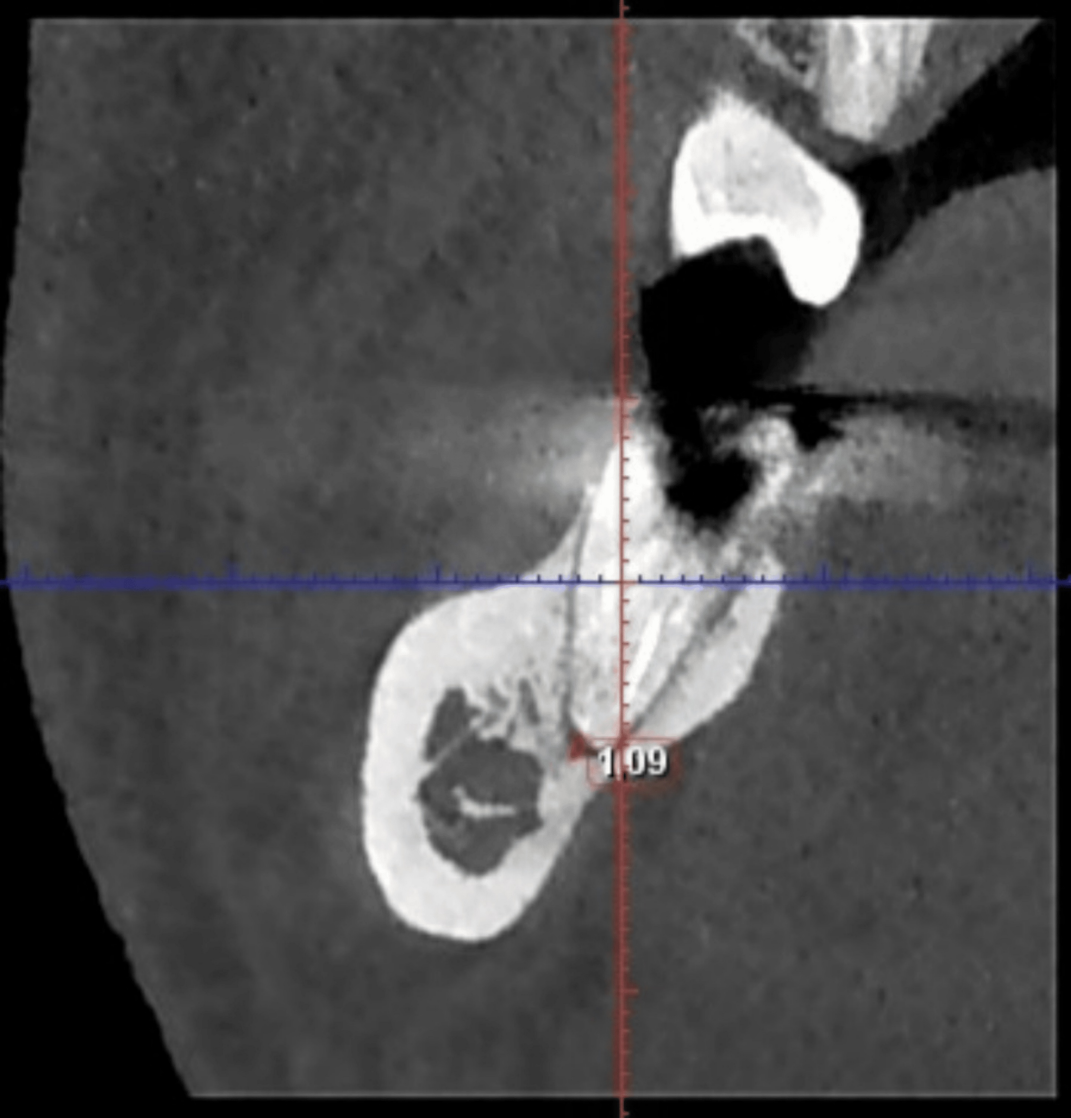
Supplementary CBCT image of the distal root of tooth 47 depicting a different measurement of the apical defect in another coronal section. CBCT, cone beam computed tomography.
